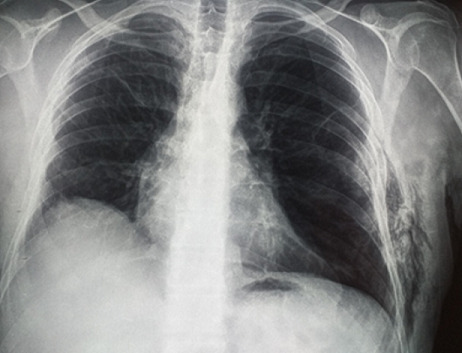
Chest X-ray: parietal air image
